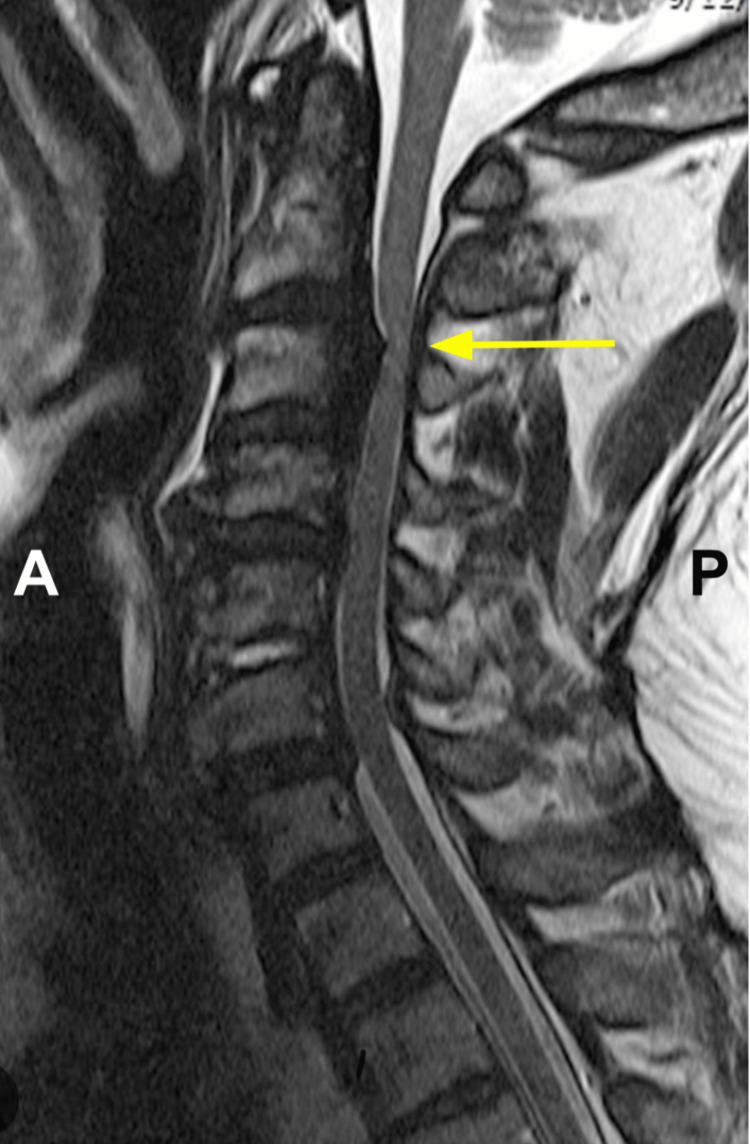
Sagittal view of preoperative cervical T2-weighted MRI. An ossified posterior longitudinal ligament is seen causing severe stenosis of the spinal canal at the level of C3-C4 vertebral bodies (yellow arrow). No T2-weighted hyperintensities noted in preoperative imaging. A: Anterior, P: Posterior
